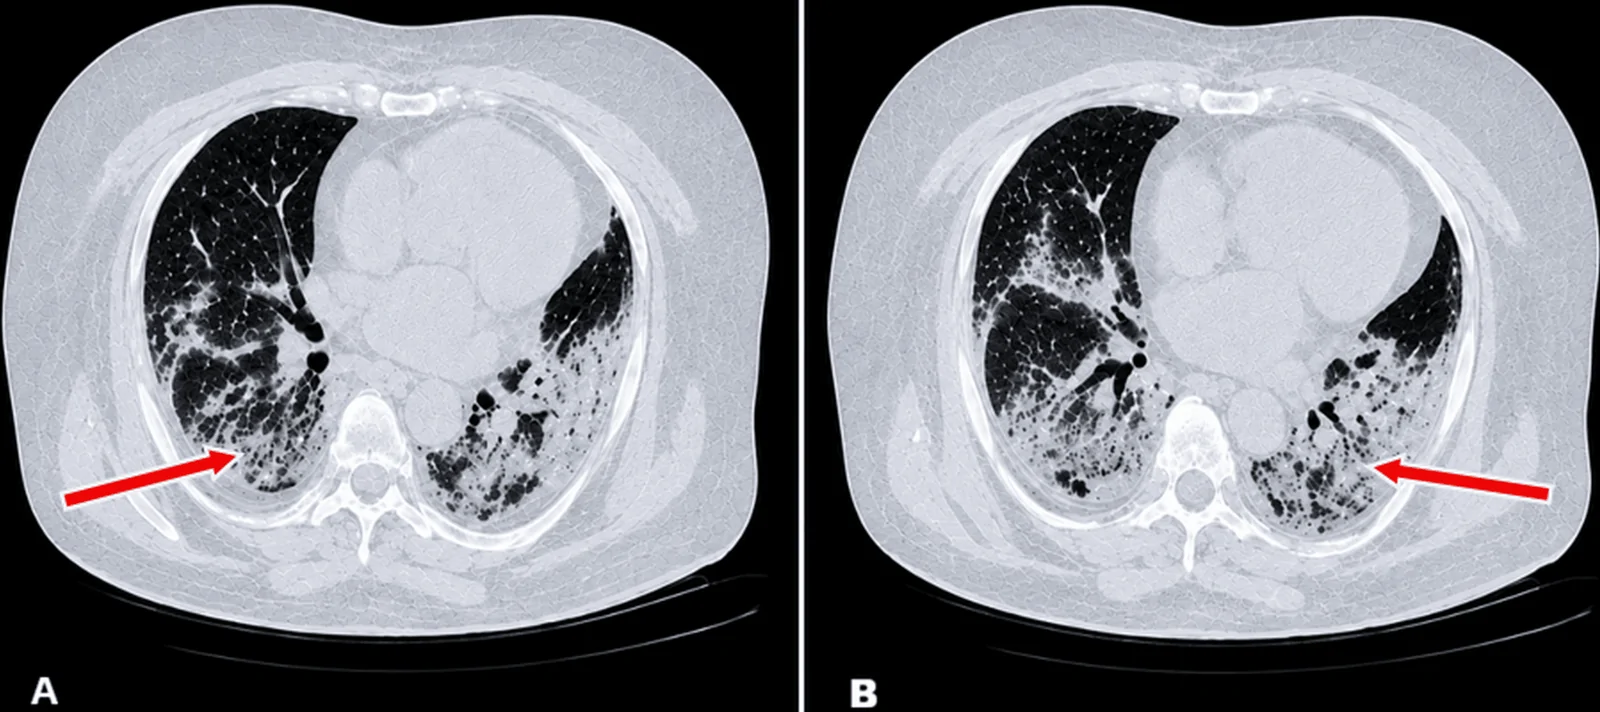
Repeat high-resolution computed tomography (HRCT) of chest showing extensive progression of lung injury Axial HRCT images obtained at different levels demonstrate bilateral diffuse ground-glass opacities with patchy consolidation and traction bronchiectatic changes (arrows), consistent with progressive diffuse lung injury.
